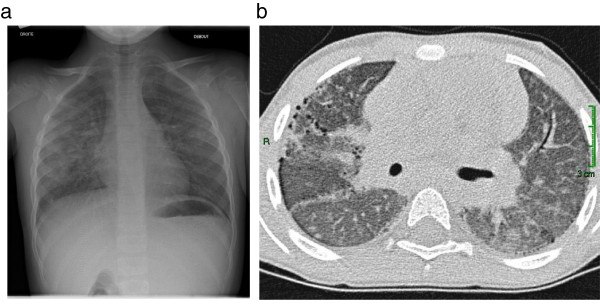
Thoracic imaging. a shows ground-glass opacities on a chest X-ray in a 5 yrs old IPH patient. b shows on a chest CT-scan an interstitial pattern with micronodules and ground-glass opacities in a 5 yrs old IPH patient.
